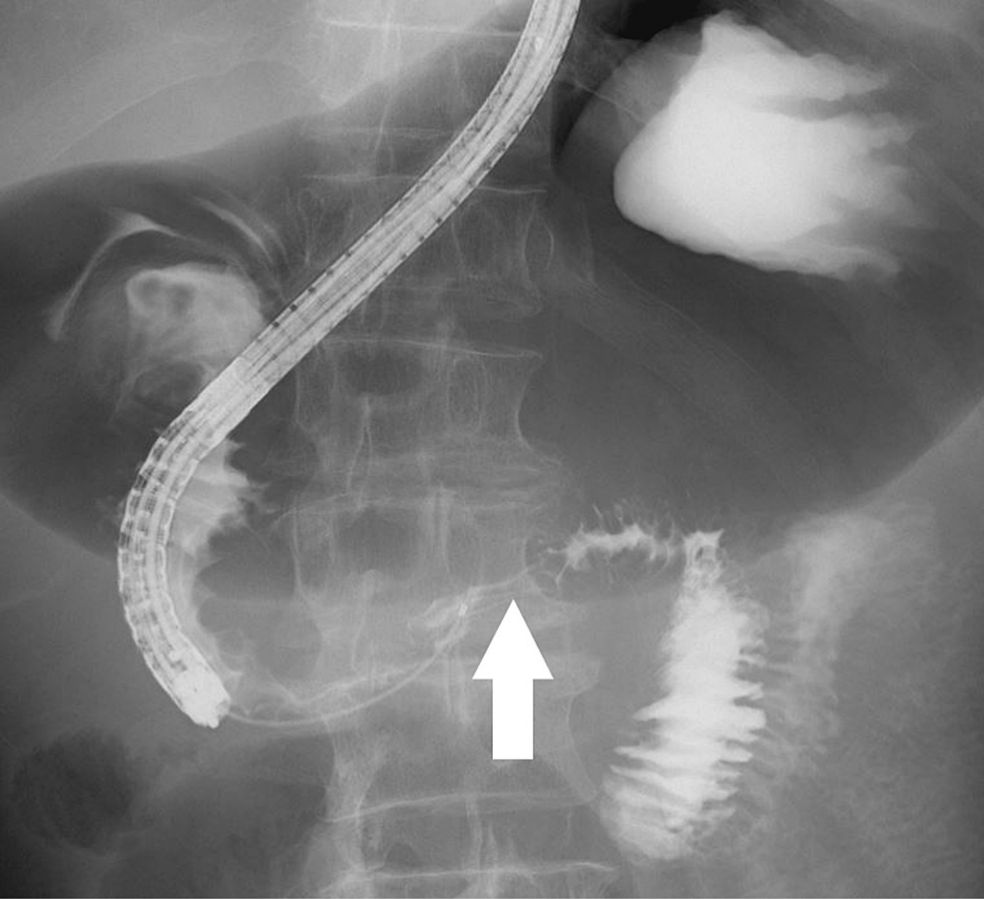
Abdominal X-ray. Gastrografin enema showed the stenosis in the third portion of the duodenum (white arrow).
